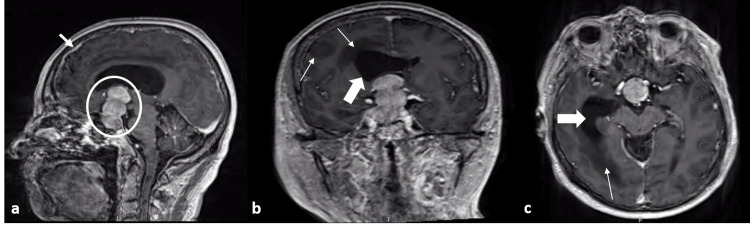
Imaging findings of case one. Magnetic resonance imaging reconstruction in T1 shows meningeal hyperintensity (arrow in a) and multiple hypointensities (thin arrows in b) in the parenchyma predominant in the right lobe. In the sella turcica, a homogeneous hyperintense mass with three well-rounded lobes can be observed (circle in a). It follows the medial line to the subventricular space causing ventriculomegaly (thick arrows in b and c).
